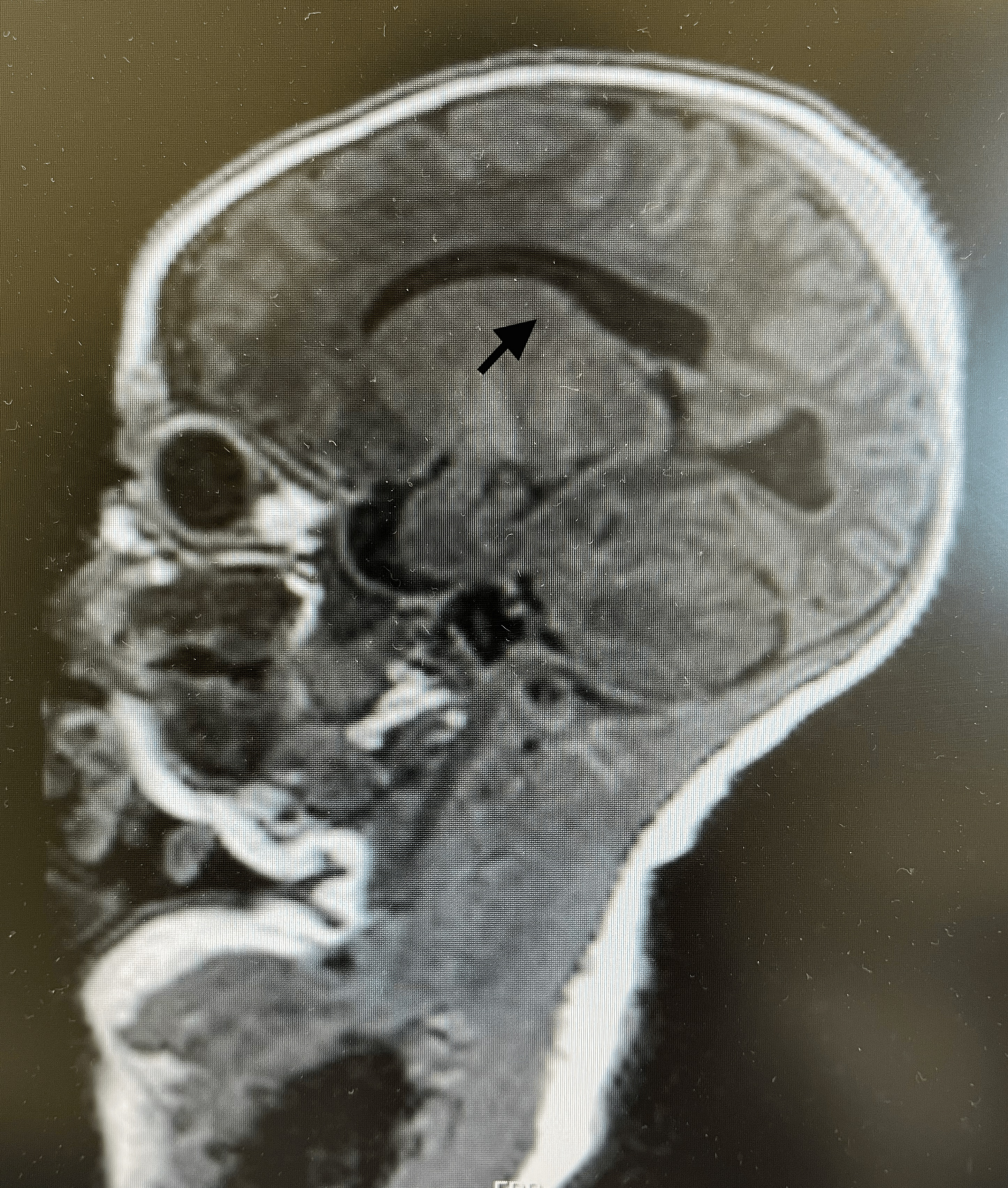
Brain MRI showing a hypoplastic corpus callosum (arrow)
